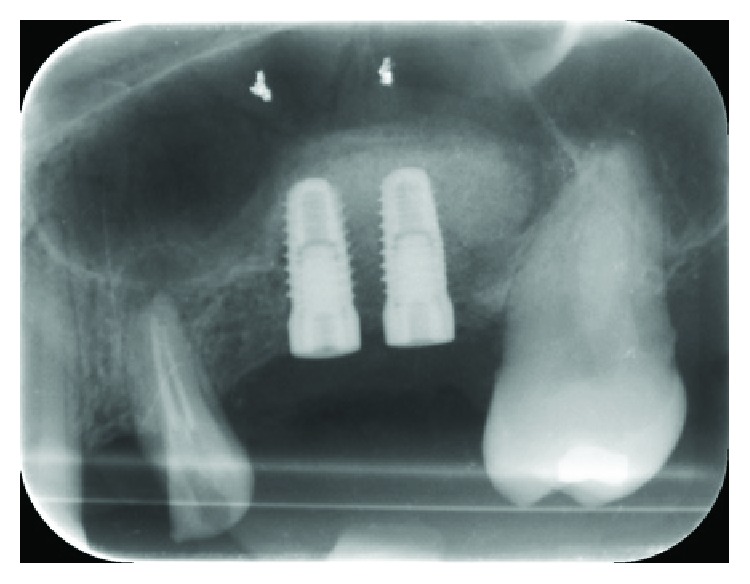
Radiograph taken postimplantation.
